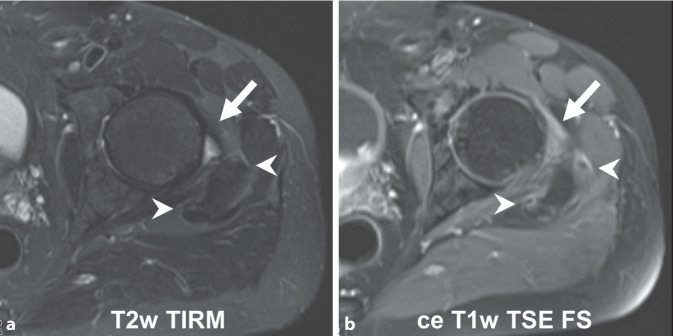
Oedema-sensitive T2-weighted turbo inversion recovery magnitude (TIRM, a) and contrast-enhanced fat-saturated T1-weighted turbo echo spin (TSE FS, b) on the level of hip joint. Pericapsular inflammation (*arrow*) as shown by contrast-enhanced magnetic resonance imaging (ceMRI) lacks a correlate by oedema-sensitive imaging. Peritendinous inflammation around glutaeus medius and minimus tendons (*arrow heads*) correlate with only minute oedema, which can easily be overlooked
